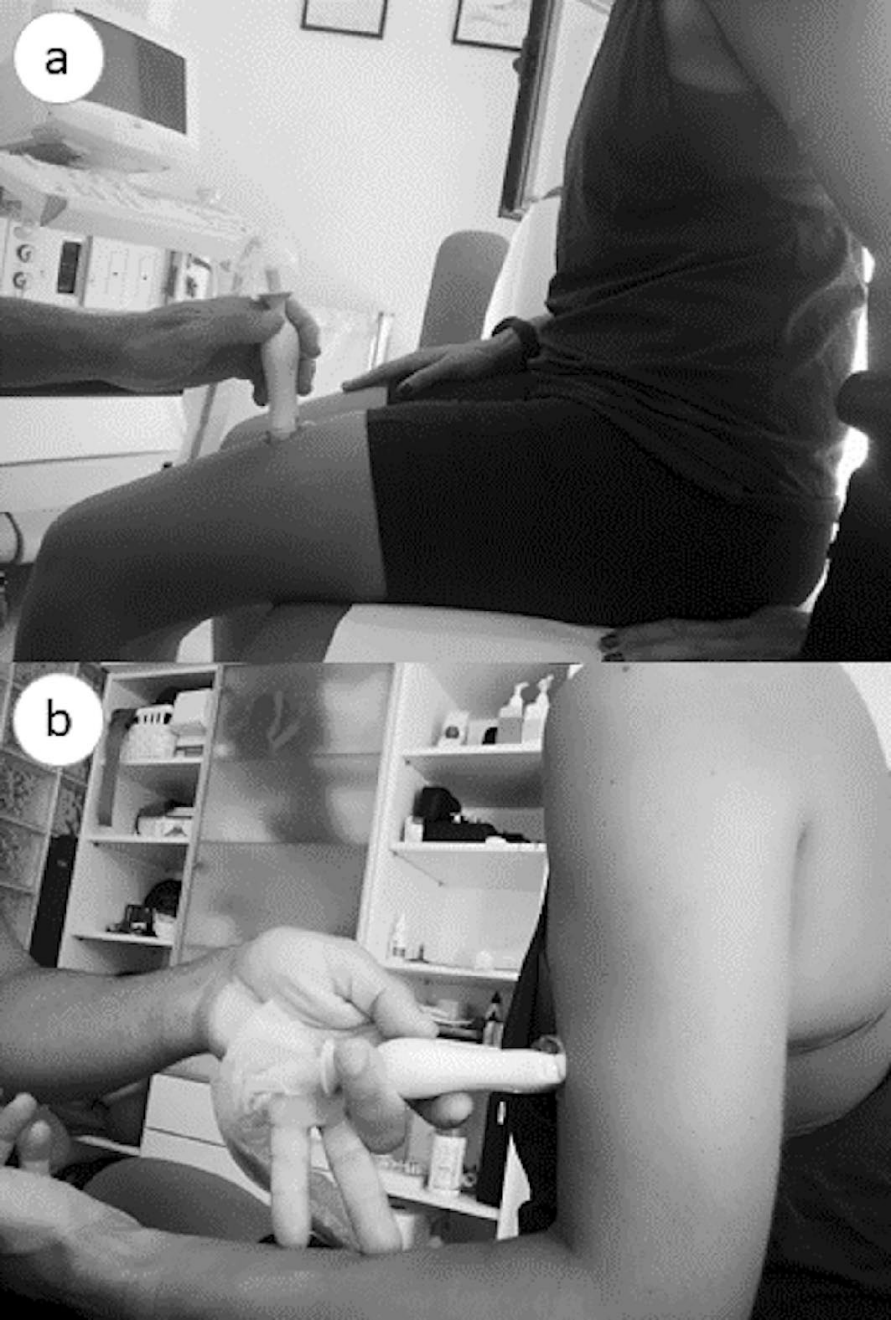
Position of the patient and the ultrasound device for ultrasound assessment of the quadriceps (a) and the biceps brachii and brachialis (b).
